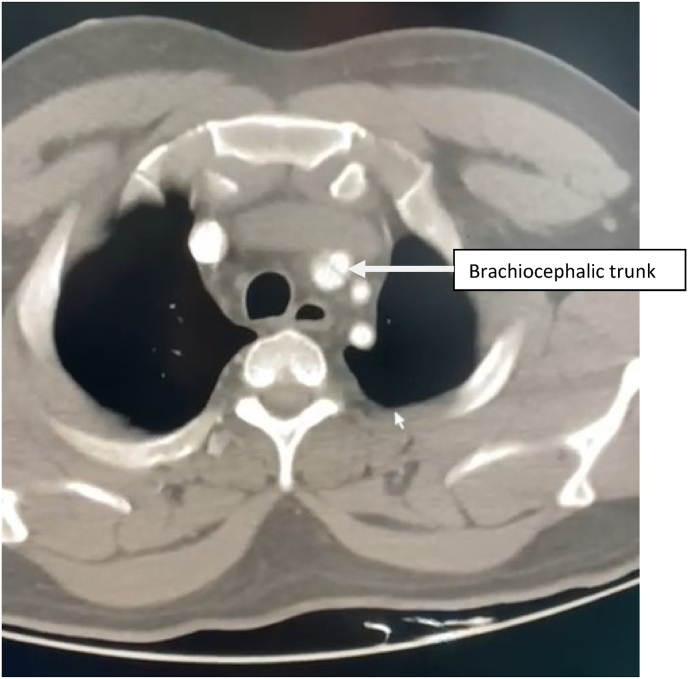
CT scan image showing the aortic dissection extending to the brachiocephalic trunk.
